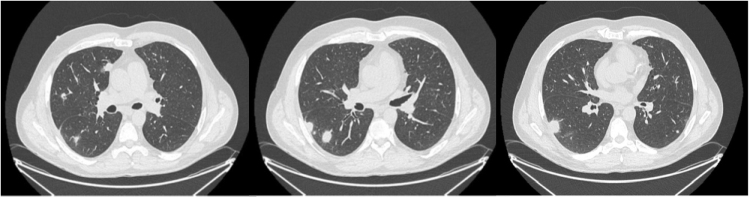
Patient’s chest CT-scan – first consultation.
